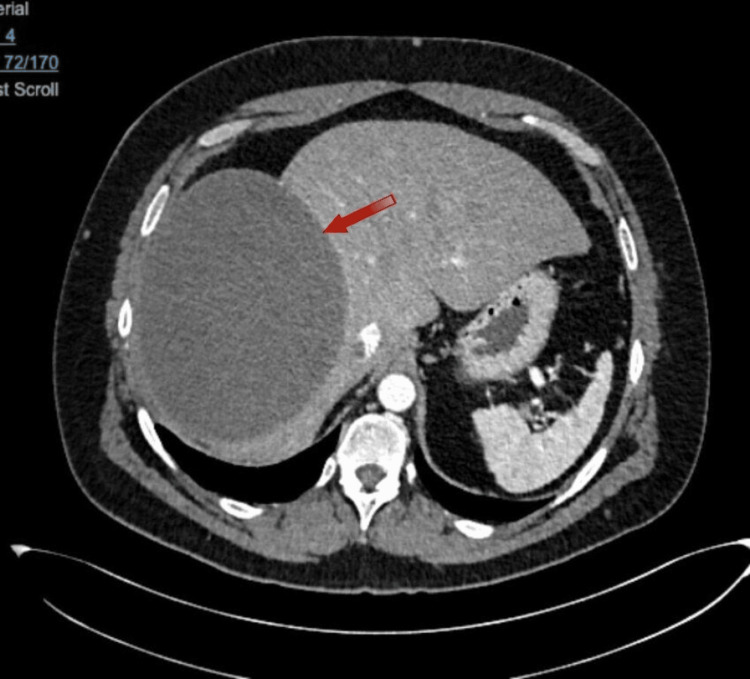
CT axial plane There is a presence of a giant, uncomplicated cyst in the right lobe.
